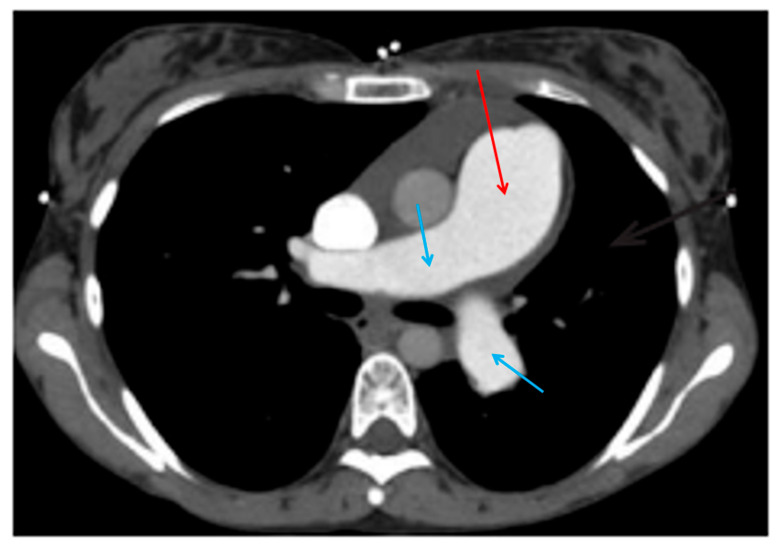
Computed tomography angiography highlighting pulmonary artery dilatation (red arrow) and dilatation and vessel widening of downstream vasculature (blue arrows) due to increased pulmonary arterial pressures in the pathological state of pulmonary hypertension. Reprinted/adapted with permission from Ref. [82].
